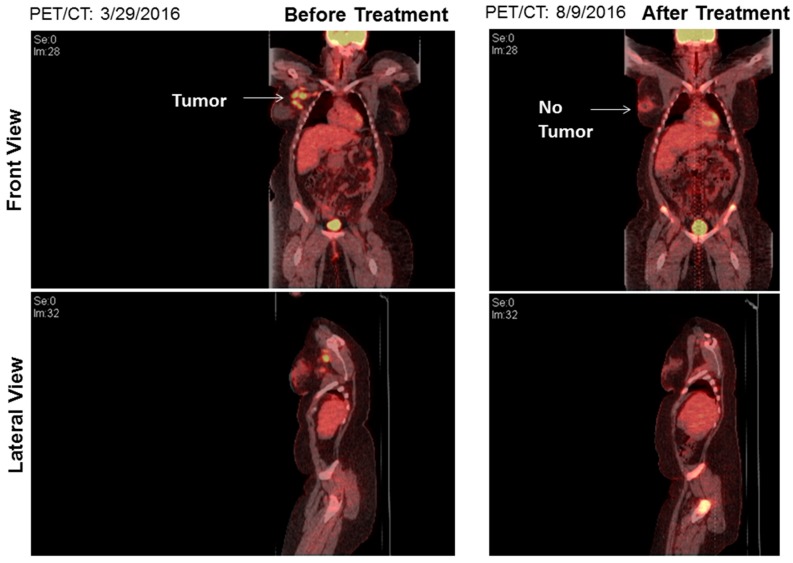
PET/CT of the tumor before (left panel) and after (right panel) the treatment. The right panel shows no evidence of tumor.
